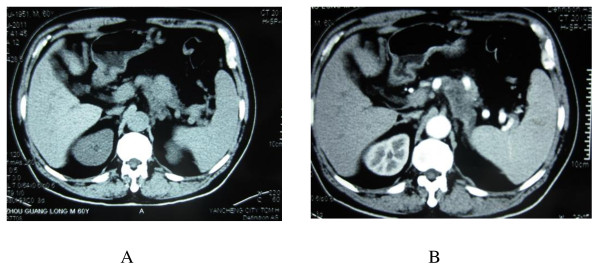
Computed tomography (CT) scan of the upper abdomen. There was a homogeneous mass in the pancreatic body and tail by noncontrast CT scan (A). In the arterial phase the mass was in low density (B).
